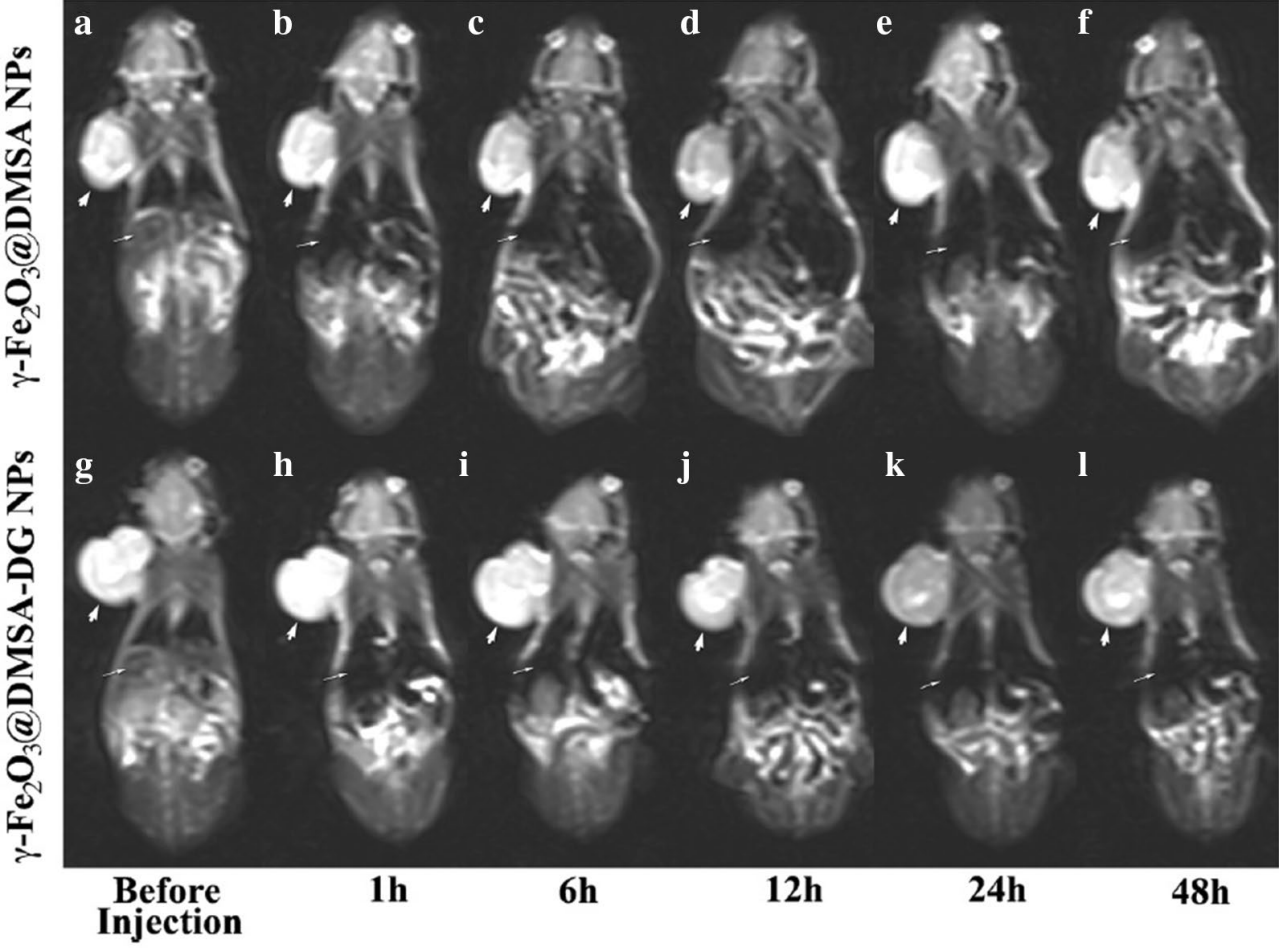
1.5-T MRI turbo-spin-echo-T2-weighted (5500/100) dynamic imaging of human MDA- MB-231 breast cancer xenografts. a–f Injection of γ-Fe_2_O_3_@DMSA NPs showed that the tumor (*thick white arrows*) signal intensity decreased at 12 h which returned to basal levels by 24 h. g–l Injection of γ-Fe_2_O_3_@DMSA-DG NPs showed that the tumor signal intensity decreased between 12 and 48 h, with the most hypointensity observed at 24 h. The signal intensity in the liver (*thin white arrows*) significantly decreased after injection of γ-Fe_2_O_3_@DMSA NPs or γ-Fe_2_O_3_@DMSA-DG NPs [48]
